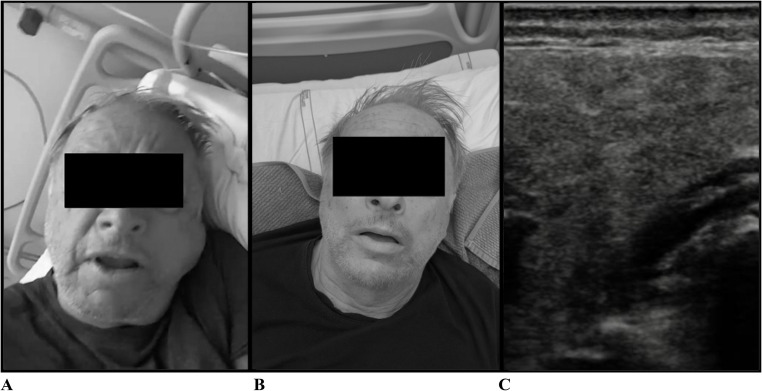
(A) Presentation of localized CRS (L-CRS) (B) clinical resolution of L-CRS after single bolus of corticosteroid therapy (C) mild parotid parenchymal hypoechogenicity on salivary gland ultrasound (B-mode)
